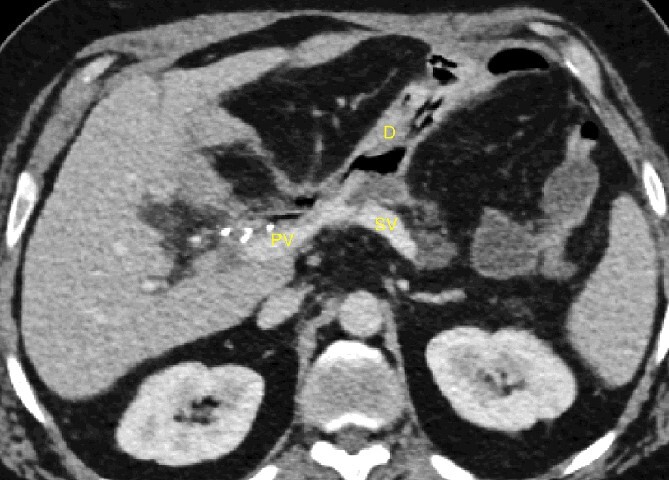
20-day post-operative CT scan showing irregularity of the splenic vein, without active bleeding. PV portal vein, SV splenic vein, D multi-tubular drain.
